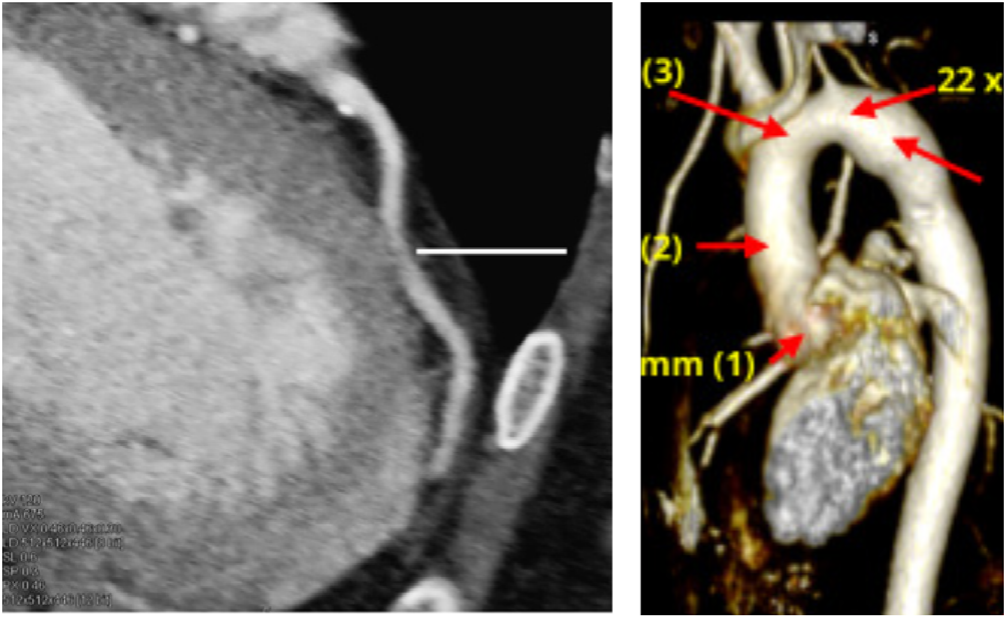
Anatomical images of blood vessels. Coronary arteries via CTA^40^ (left) and Coarctations of the aorta via MRA after thresholding non‐blood tissues^41^ (right). Reprinted with permission
